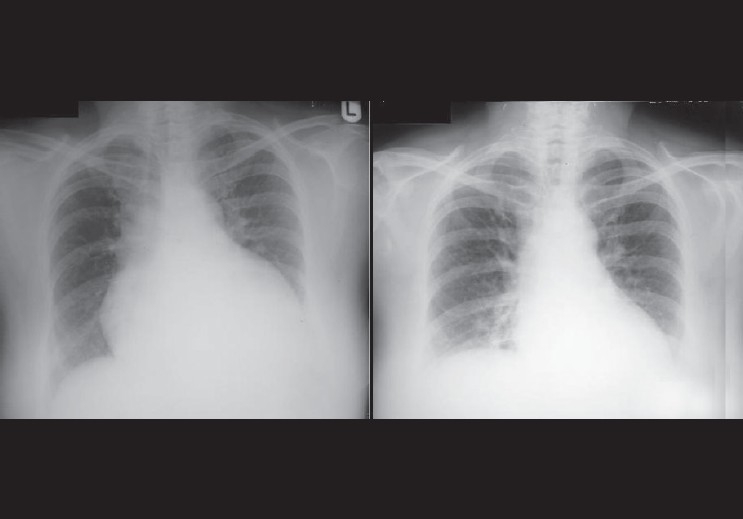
The radiograph on the left was taken on admission of the patient, showing an enlarged globular heart secondary to pericardial effusion due to severe hypothyroidism. The image on the right was taken 3 weeks later, showing resolution of the pericardial effusion
